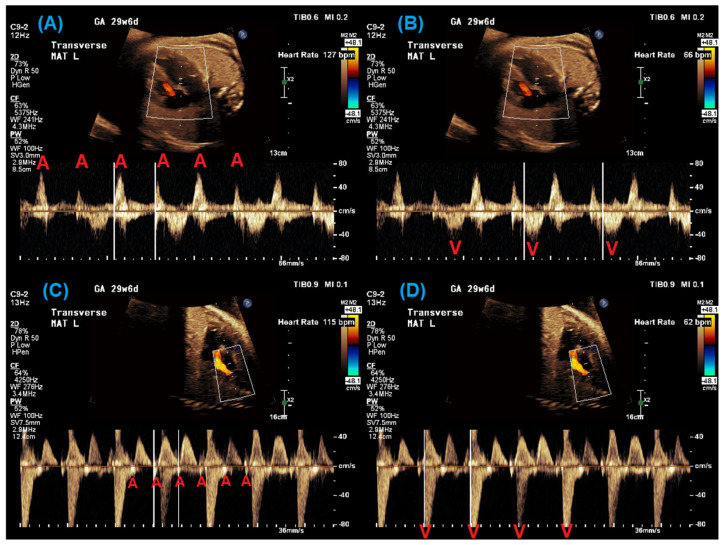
Pulsed-wave Doppler evaluation of a 29-week fetal patient with 2:1 AV block. From left to right: The evaluation of mitral valve inflow–aortic valve outflow Doppler with atrial rate (A) and ventricular rate (B) measured and superior vena cava–aorta Doppler with atrial rate (C) and ventricular rate (D) measured. Red A = atrial beat; red V = ventricular beat.
